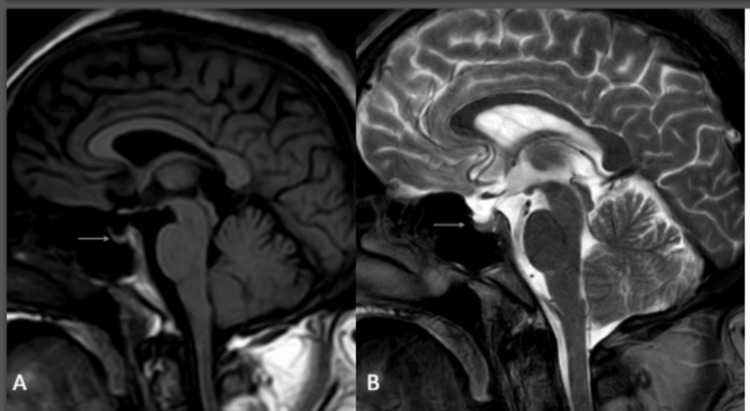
MRI brain sagittal section MRI: Magnetic resonance imaging (A) T1 weighted and (B) T2 weighted showing a partially empty sella (white arrows)
